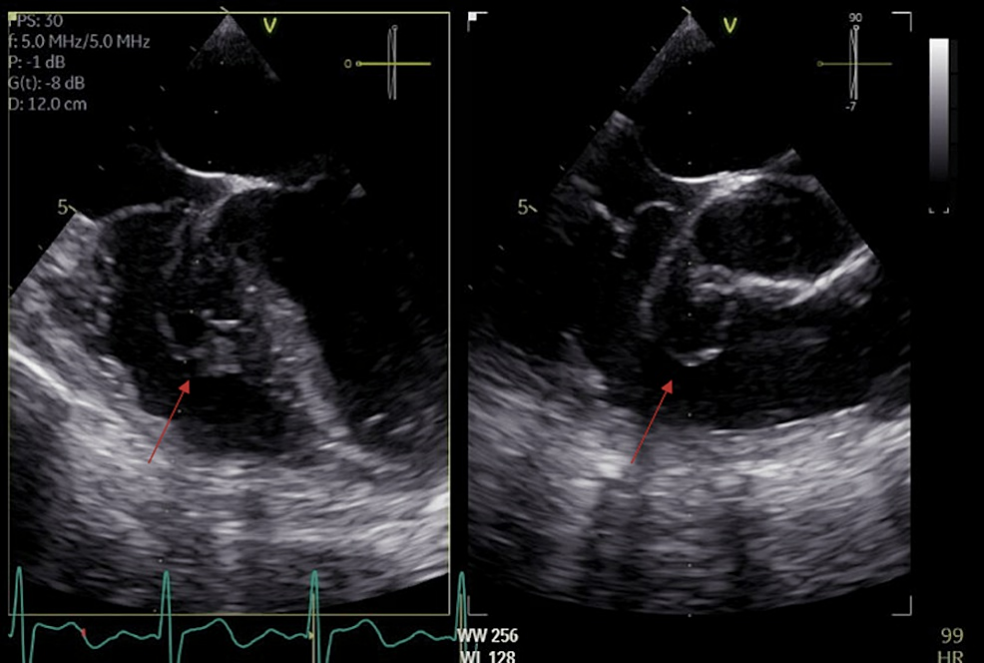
TEE images of the VSD patch in the four-chamber view with anterior and posterior views. TEE, transesophageal echocardiogram; VSD, ventricular septal defect
